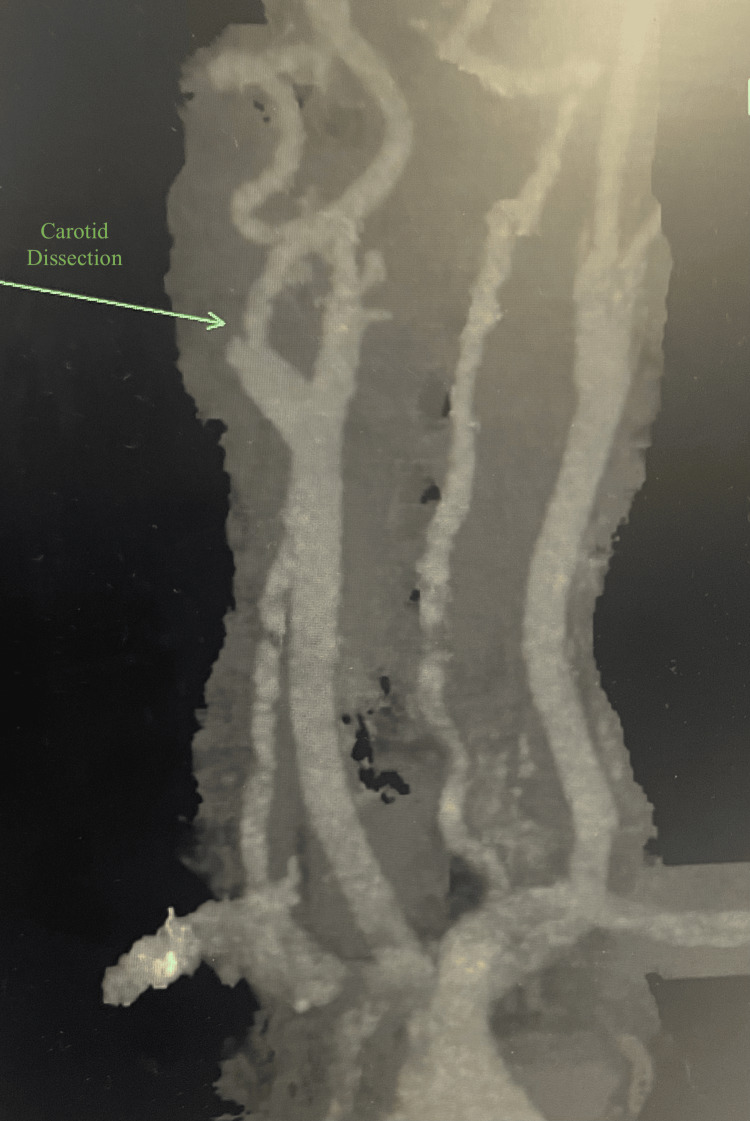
CTA of the neck showing right-sided carotid dissection (arrow) CTA: computed tomography angiography
